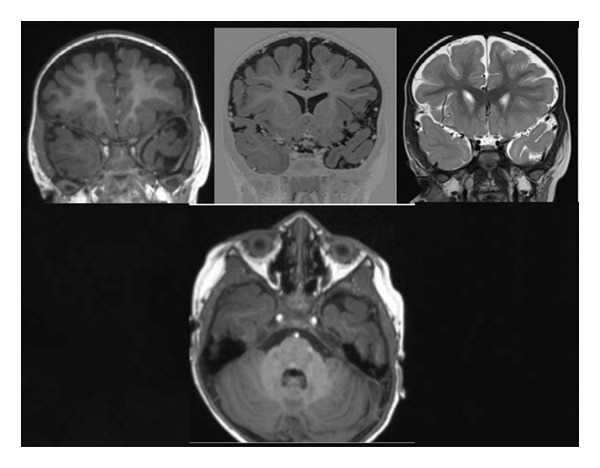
Coronal T1‐ and T2‐weighted MRI sequences demonstrating asymmetry of the temporal lobes. The left temporal lobe and hippocampus exhibit a mild volume reduction compared with the right side.
